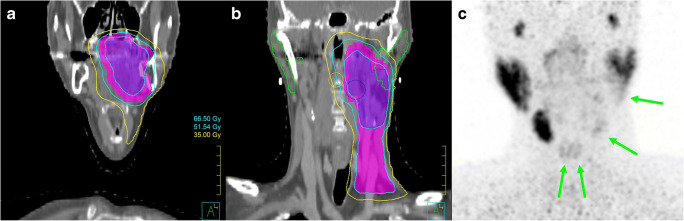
Planning CT (a, b) and 1 year post-therapy ^68^Ga-PSMA PET image (c), acquired for a patient who received RT for tonsillar carcinoma. This image was reproduced from Valstar et al. with permission [106]
